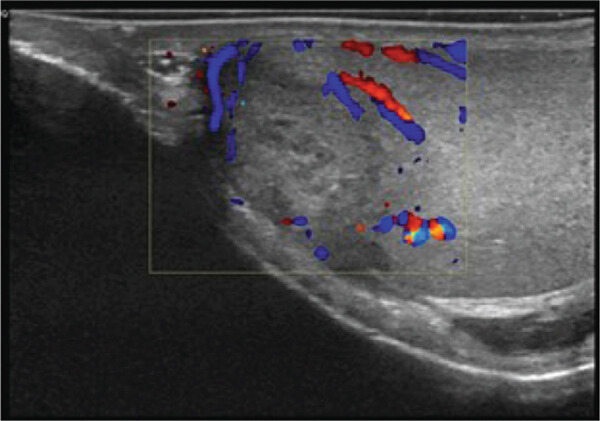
Heterogeneous lesion on ultrasound with a nodule of 2.3 × 1.4 × 1.3 cm in the right testicle [1].
